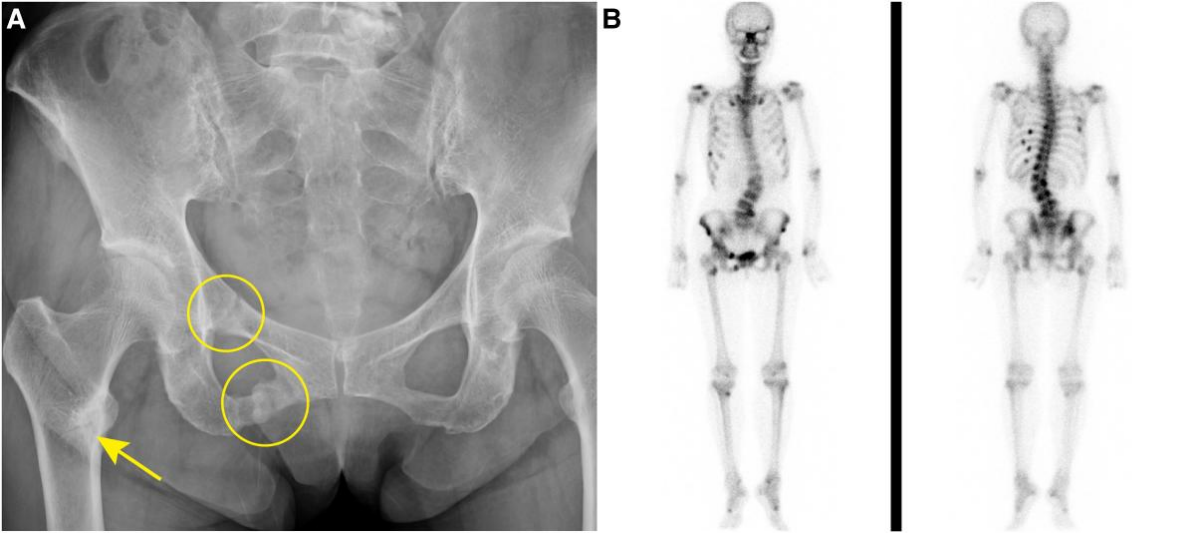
Pelvic radiography and bone scintigraphy. A, Pelvic radiography: pseudofracture of bones (Looser zone) are shown by yellow circles and a yellow arrow. B, Bone scintigraphy: There are multiple uptakes throughout the skeletal system.
